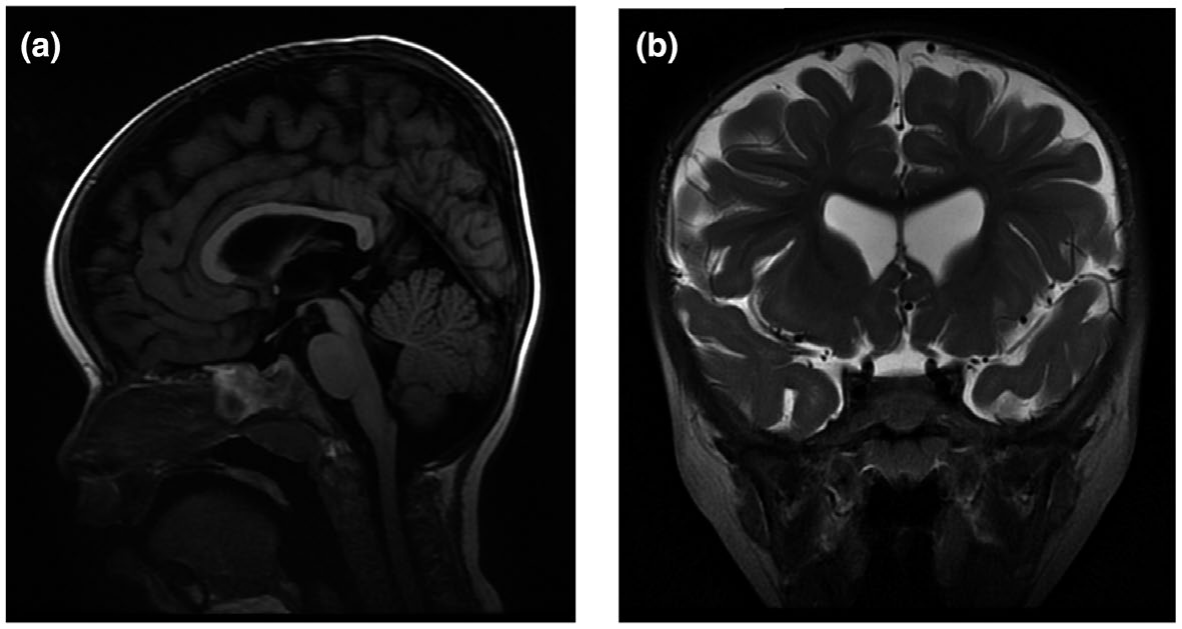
Magnetic resonance imaging of the brain. (a) sagittal and (b) coronal plane showing widened cerebral subarachnoid space, cerebral ventriculomegaly, and generalized mild cerebral cortex volume loss
